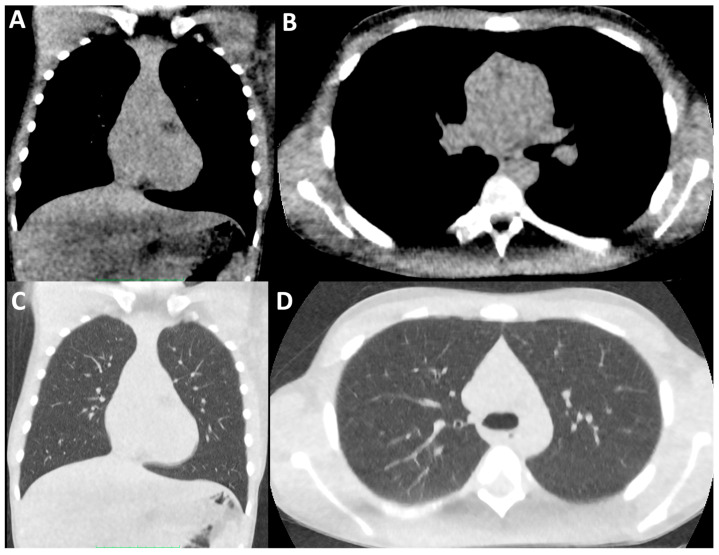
Ultra-low-dose CT of the thorax. Coronally (A,C) and axially (B,D) reconstructed ultra-low-dose CT thorax images in soft tissue (A,B) and lung windows (C,D) in the same paediatric male CF patient as Figure 1, performed during routine disease surveillance several years later. The DLP for this examination was 2.28 mGy*cm.
